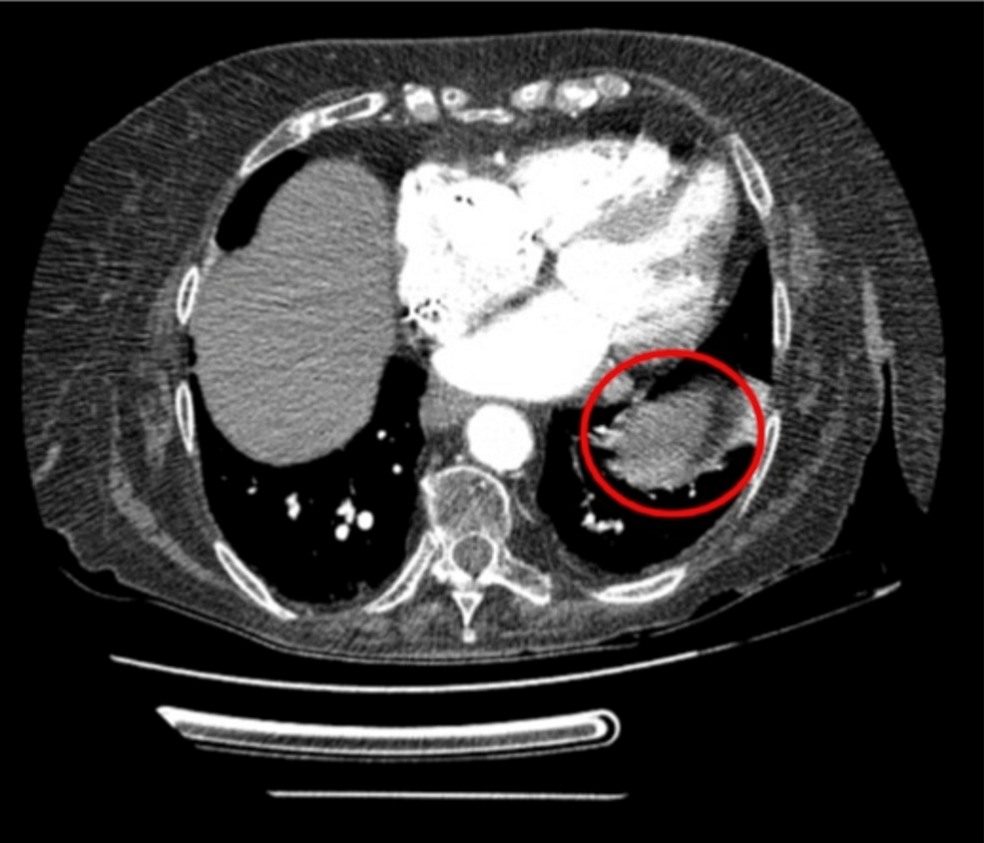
Computed tomography with angiography depicting an amorphous area of mass-like consolidation in the anterior left lower lobe at the left lung base (red circle).
